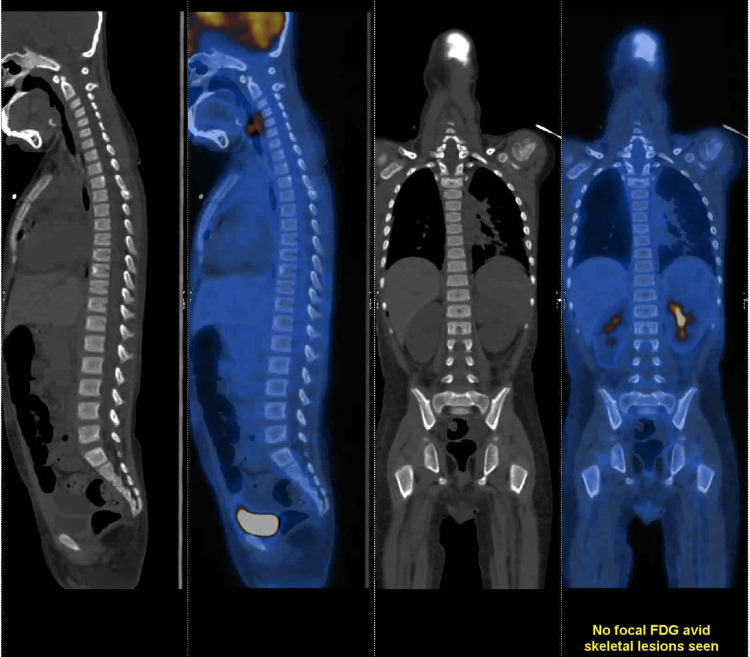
Whole-body PET scan No Fludeoxyglucose F18 (FDG) activity was observed.
